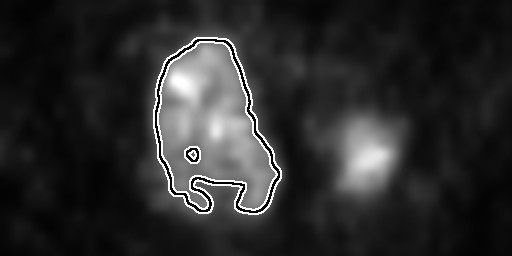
Heterogeneity in an FDG-PET image. A typical FDG-PET image of a cancer of the uterine cervix. The artificial boundary delineates the region of activity above the 40% of maximum intensity threshold. The heterogeneity within the tumor is evidenced by the very bright regions (higher metabolic activity) juxtaposed with relatively dark regions (lower metabolic activity). The undelineated bright spot to the right is a lymph node and is thus not included in the main tumor volume. The vertical edge of this image represents a length of 10 cm.
